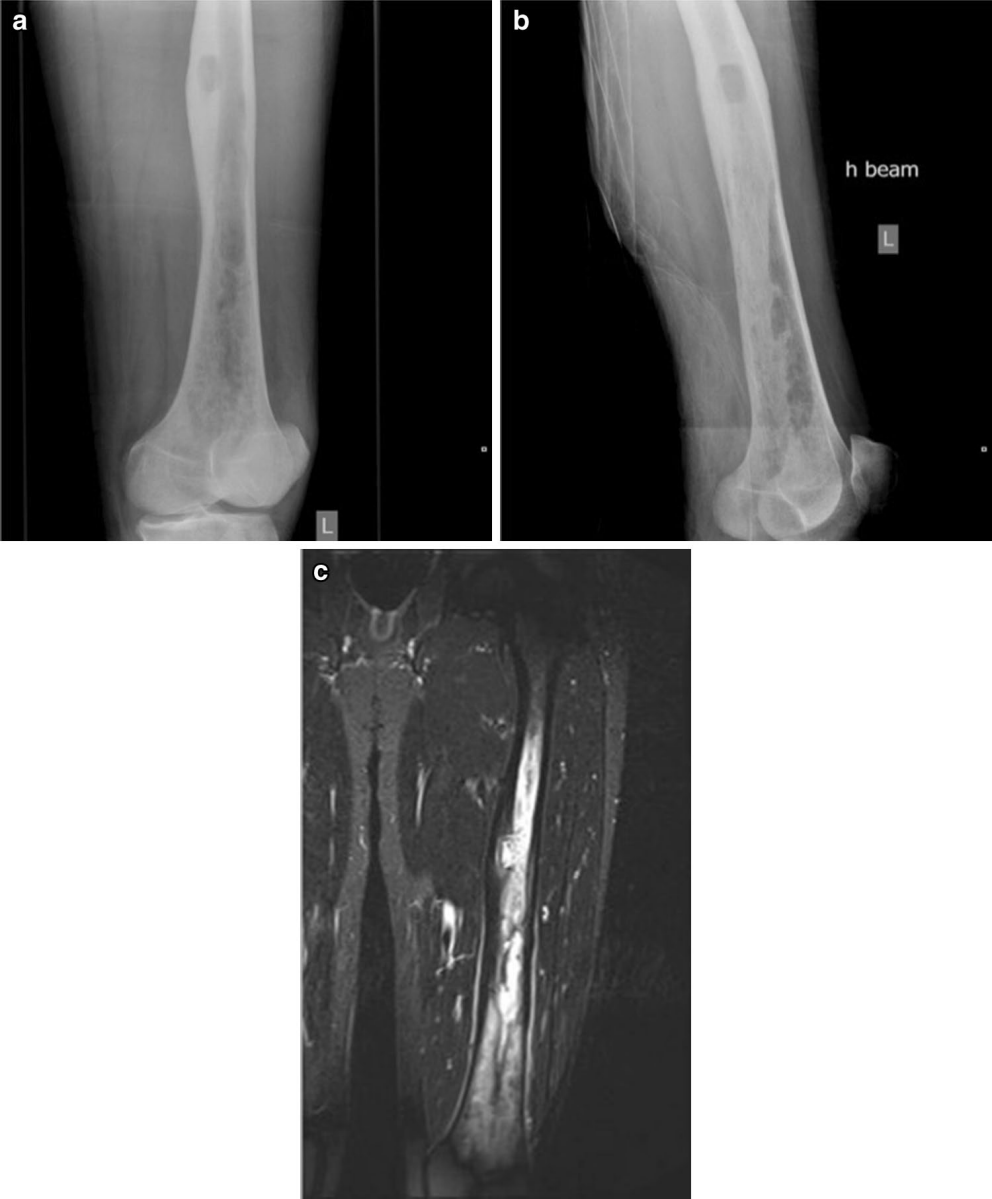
a Anteroposterior b lateral radiographs and c MRI of the left femur of a 34-year-old female patient presenting with gradual onset left thigh pain and elevated inflammatory markers, showing a native osteomyelitis
